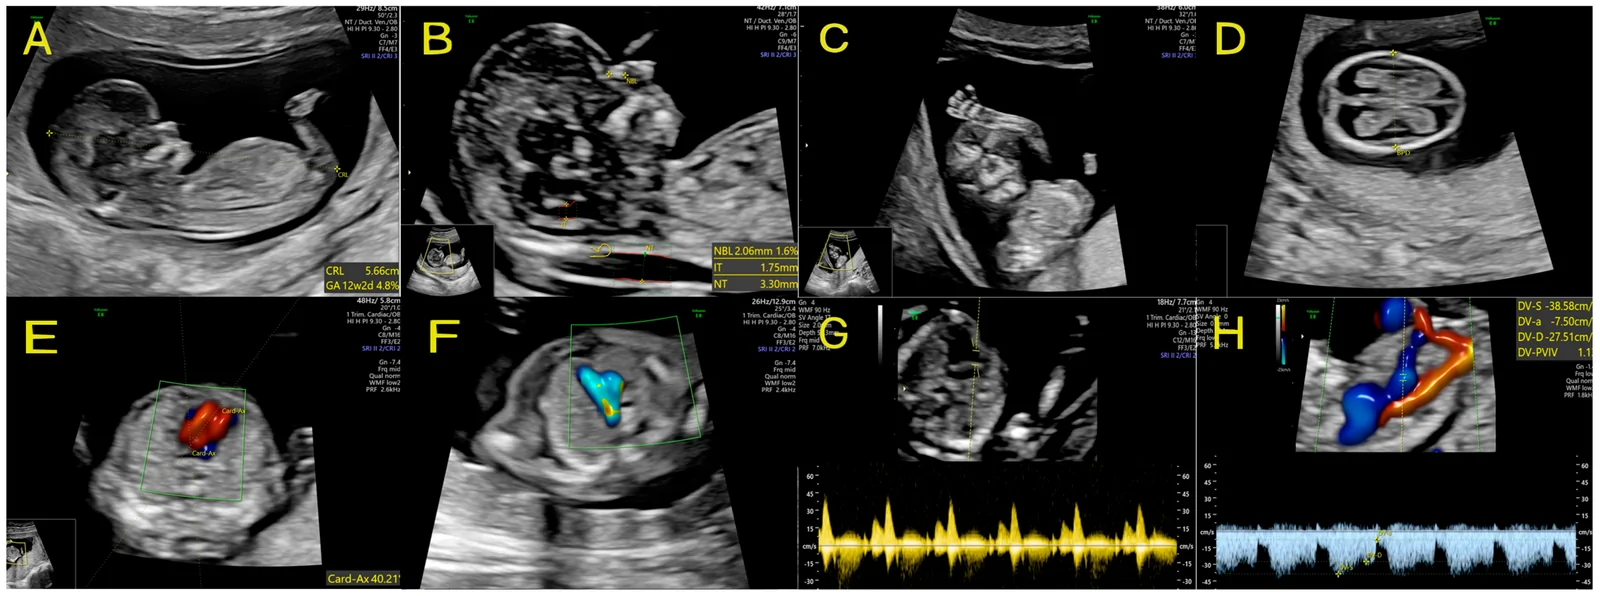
Representative ultrasound images demonstrating the first-trimester screening (11 + 0 to 13 + 6 weeks) in the anatomical protocol used in the study. (A) Crown-rump length (CRL) measurement: strict midsagittal view of the fetus in a neutral position with horizontal calipers placed from the crown to the rump. (B) Midsagittal view of the fetal face: assessment of the profile demonstrating correct magnification for nuchal translucency (NT) measurement, presence of the nasal bone (NB), and clear visualization of the intracranial translucency (IT) in the posterior fossa. (C) Retro-nasal triangle view: coronal scan of the fetal face used to evaluate the primary palate and exclude cleft lip/palate. (D) Transverse view of the brain: axial plane demonstrating the normal, symmetrical "butterfly sign" formed by the choroid plexuses within the lateral ventricles. (E) Four-chamber view (4CV) of the heart: apical projection at the level of the fetal thorax demonstrating cardiac axis symmetry and chamber balance. (F) Three-vessel and trachea (3VT) view: axial upper mediastinal plane showing the normal alignment and spatial relationship of the pulmonary artery, aorta, and superior vena cava relative to the trachea. (G) Axial plane of the fetal chest showing placement of the pulsed Doppler sample volume for tricuspid Doppler flow assessment. Color Doppler assessment of tricuspid valve flow: interrogation of ventricular inflow to confirm the absence of significant tricuspid regurgitation. (H) Parasagittal view of abdomen and chest in color Doppler assessment of ductus venosus flow: high-resolution color Doppler visualization evaluating the classic triphasic waveform profile.
